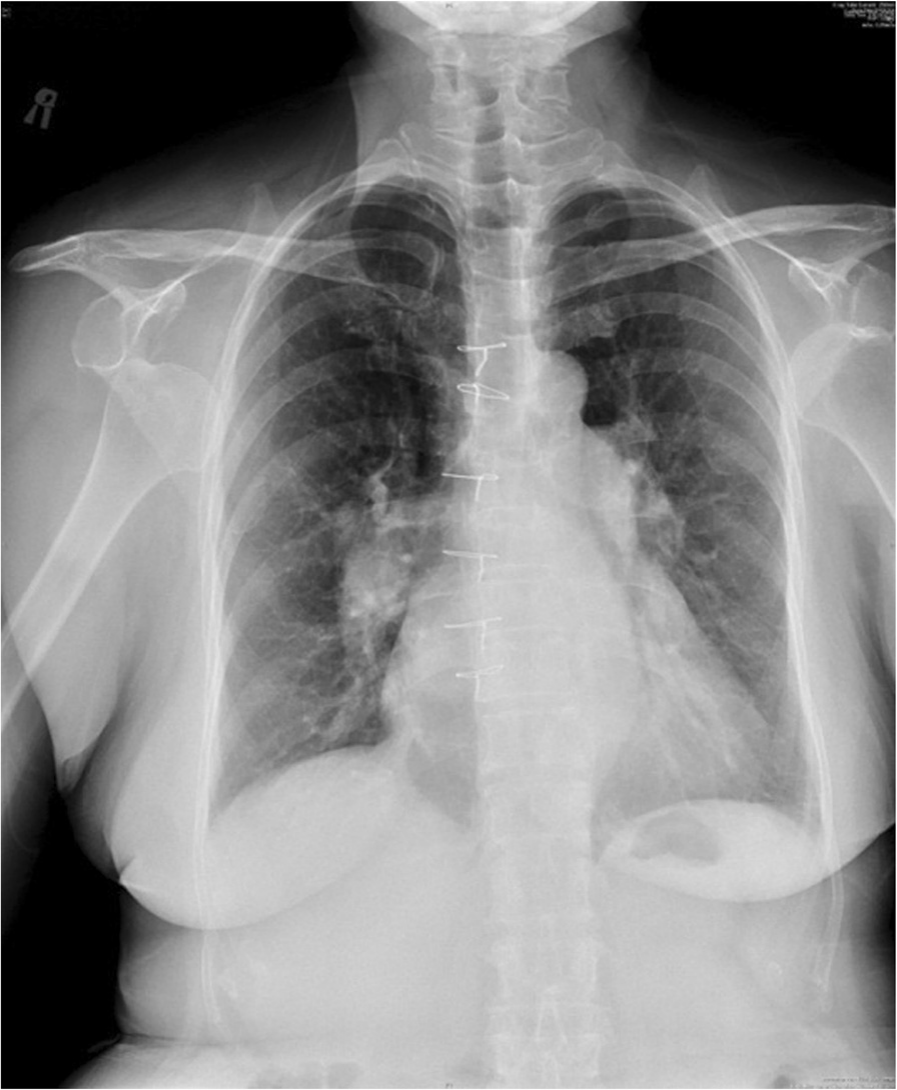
Chest radiograph demonstrating bilateral anterior shoulder dislocations.
